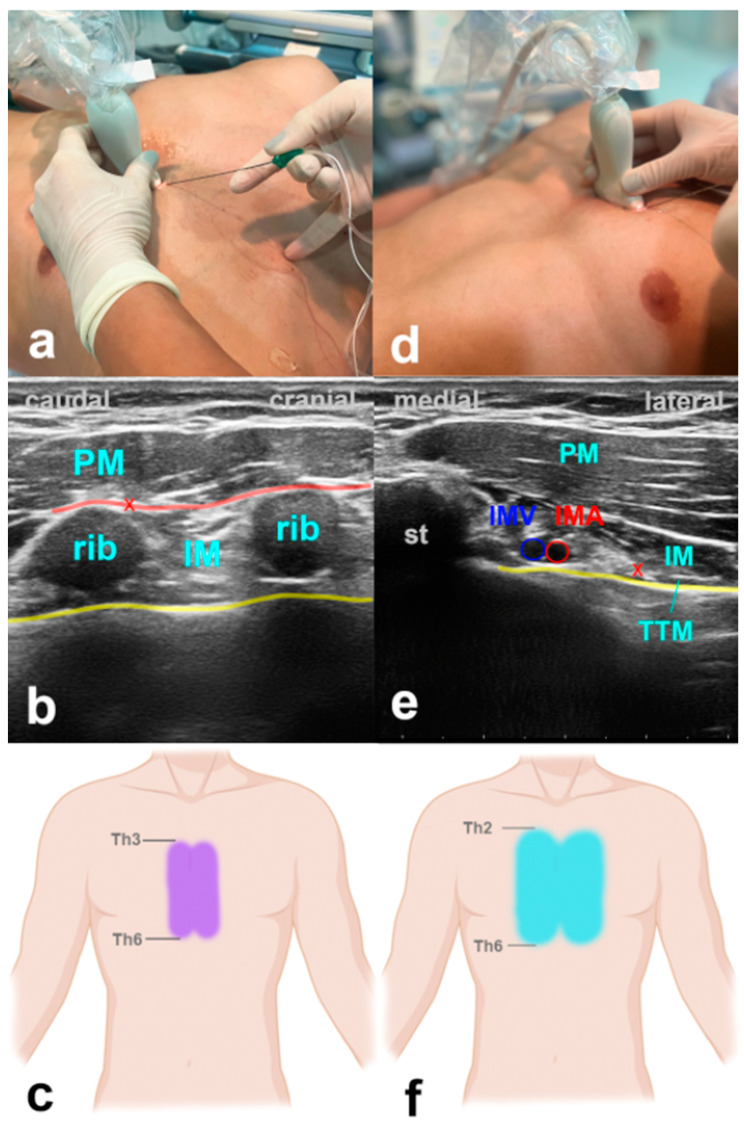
Parasternal blocks. (a) Transducer positioning for PIFB. (b) Ultrasound anatomy of PIFB: PM—pectoralis muscle; IM—intercostal muscle; yellow line—pleura; red cross—target point for the injection at the edge of the rib; red line—target space for PIFB. (c) Spread of PIFB. (d) Transducer positioning for TTPB. (e) Ultrasound anatomy of TTPB: PM—pectoralis muscle; IM—intercostal muscle; TTM—transverse thoracic muscle; st—sternum; IMV—internal mammary vein; IMA—internal mammary artery; yellow line—pleura; red cross—target point for the injection between IM and TTM. (f) Spread of TTPB.
